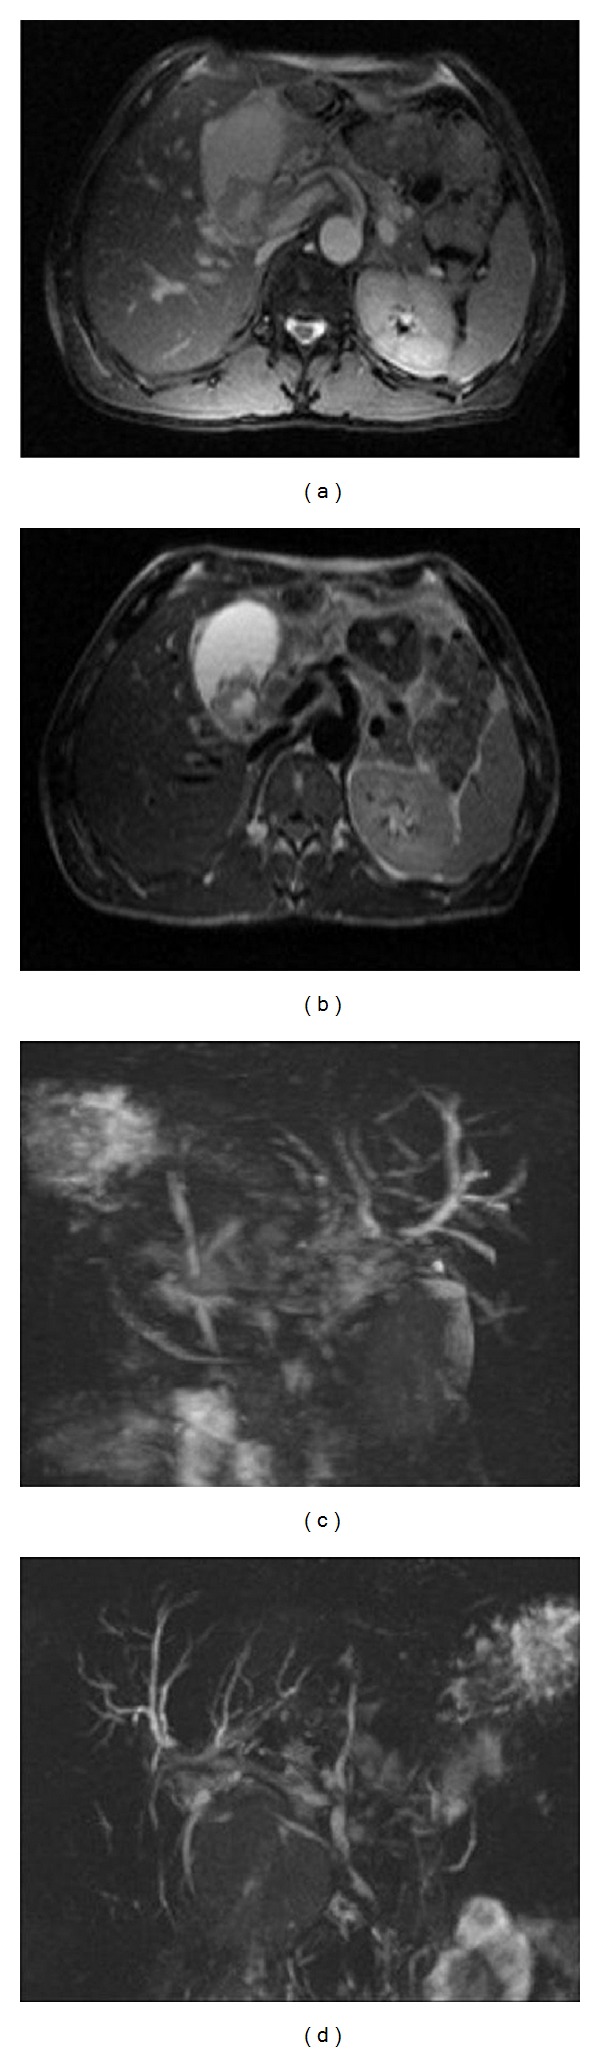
(a), (b) Axial fat saturated T2-weighted magnetic resonance images showing bile ducts dilatation and a fluid collection around left hepatic lobe and around gallbladder; inside the main bile duct and in the gallbladder note materials with hypointense signal due to blood clots. (c), (d) 2D and 3D haste MIP images showing low signal intensity of blood clots inside dilatated bile tree, sign of haemobilia, and inflammatory narrowing of distal choledocus.
